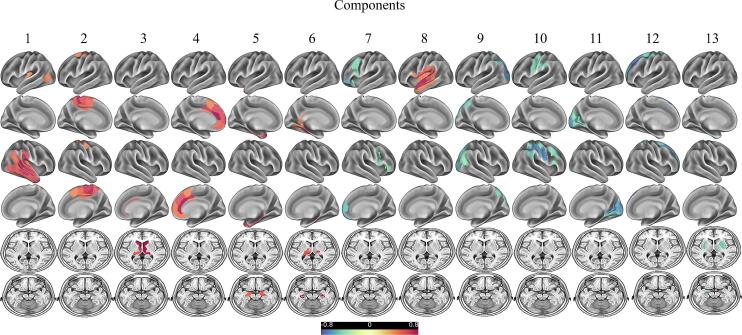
Principal components of [^18^F]-PI-2620 SUVr z-scores. Rotated regional weights of [^18^F]PI-2620 SUVr z-score components identified by applying principal component analysis on 246 cerebral regions. The colours represent the region-specific weights (range from −0.8 to 0.8) on each component.
